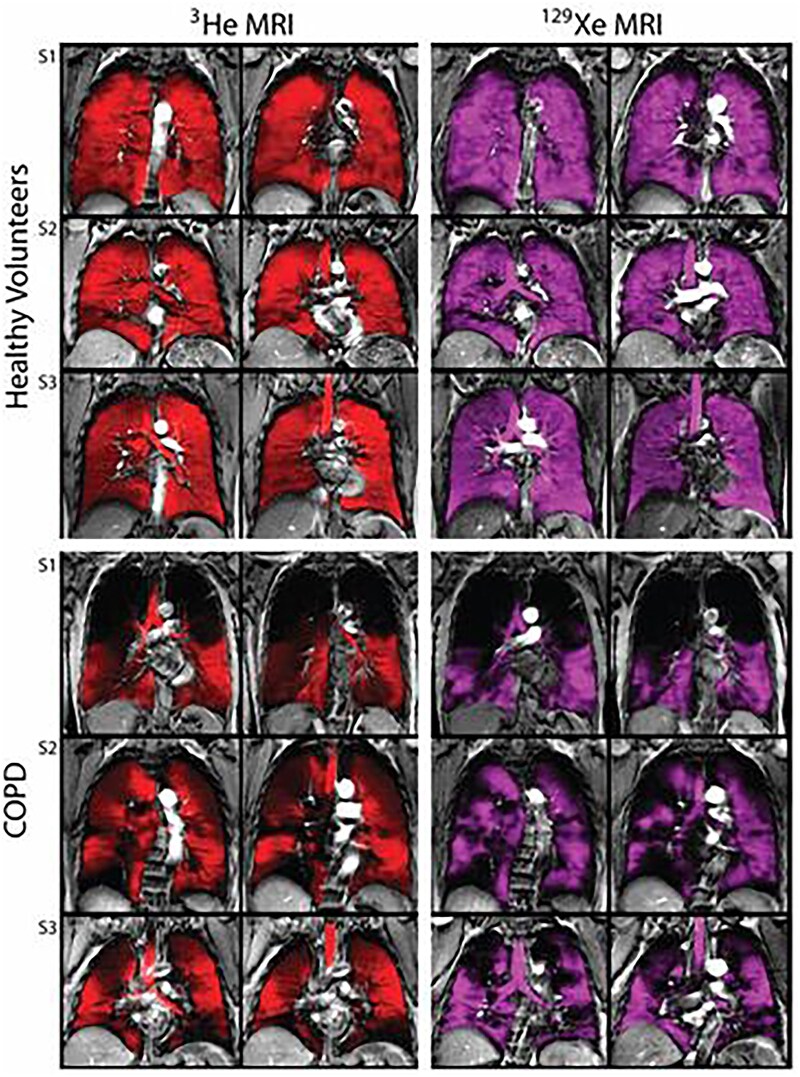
Static ventilation 3He and 129Xe MR images in 3 healthy volunteers and 3 patients with COPD. Images of the 2 coronal center sections, where trachea and 2 main bronchi are clearly visible, are registered to greyscale 1H MR images of thorax. Volunteer in S1 is 75-year-old woman with percentage predicted FEV1 of 93% and FEV1/FVC of 70%; volunteer in S2 is 57-year-old man with percentage predicted FEV1 of 95% and FEV1/FVC of 72%; volunteer in S3 is 51-year-old man with percentage predicted FEV1 of 120% and FEV1/FVC of 83%. Patient in S1 is 77-year-old woman with percentage predicted FEV1 of 50% and FEV1/FVC of 20%; patient in S2 is 68-year-old woman with percentage predicted FEV1 of 59% and FEV1/FVC of 53%; patient in S3 is 71-year-old man with percentage predicted FEV1 of 107% and FEV1/FVC of 58%. 3He gas distribution is displayed in red and 129Xe gas distribution is displayed in purple. Reproduced with permission from Kirby et al, Academic Radiology, 2012; 19(2):141-52. © 2012 Elsevier.^30^
